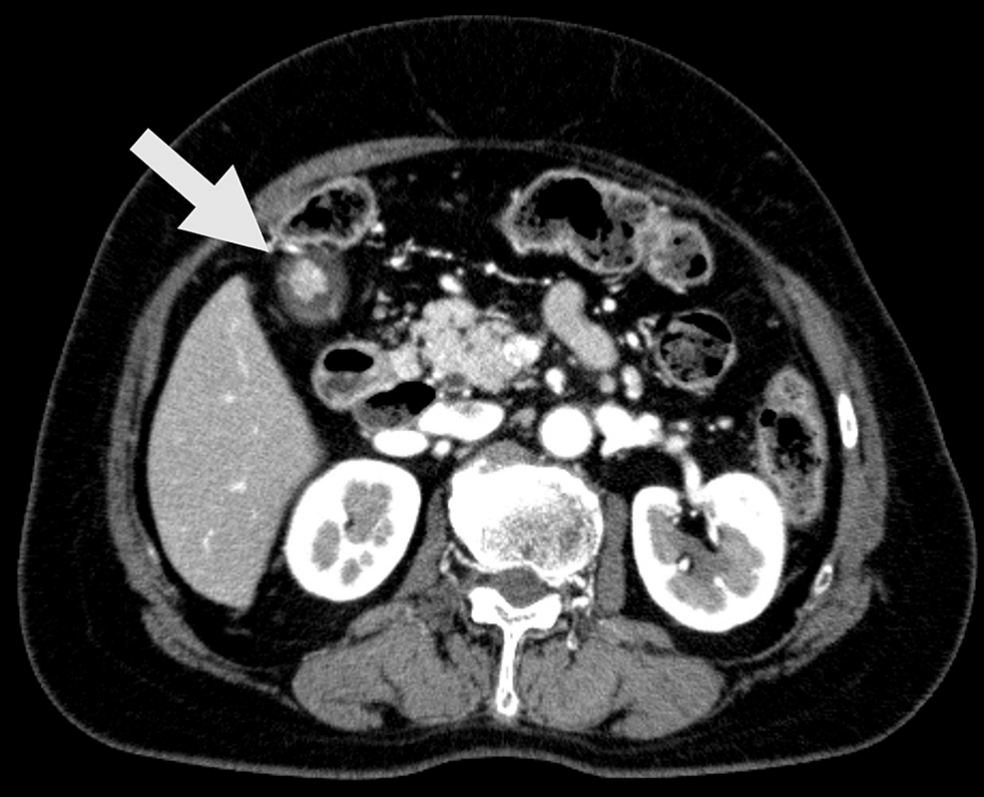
Abdominal contrast-enhanced computed tomography The arterial phase of abdominal contrast-enhanced computed tomography revealed a lobulated polyp 10 mm in size in the fundus of the gallbladder as a hyper-enhanced lesion.
